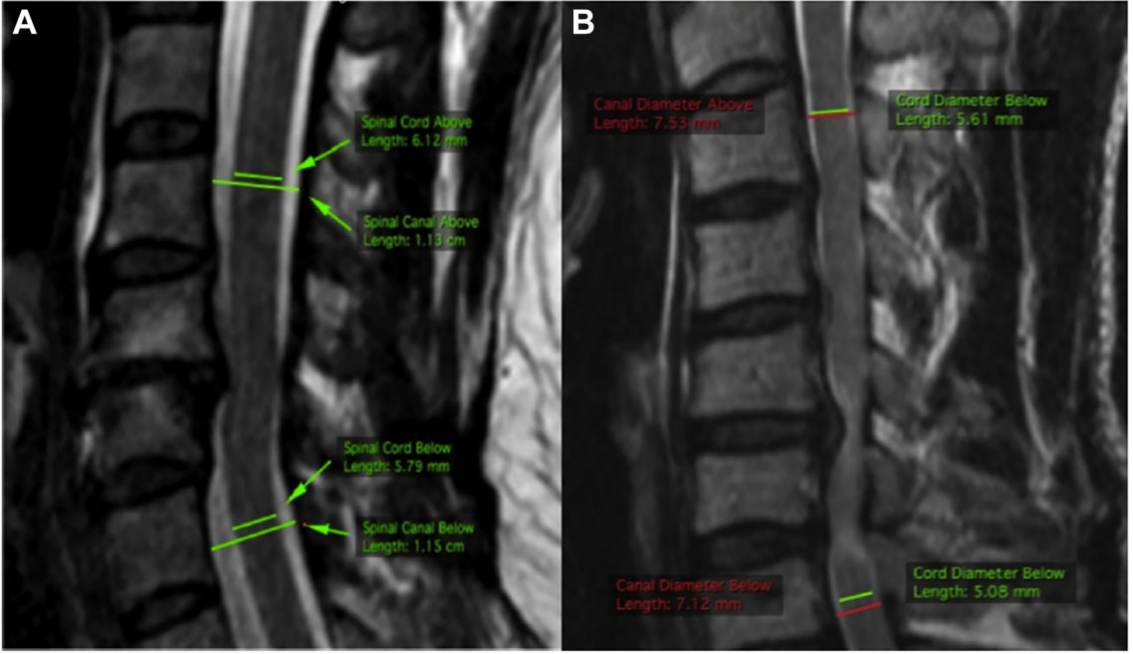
Cord-Canal mismatch measurement in 2 different patients based on sagittal T2 MRI. A, Represents a patient without a cord-canal mismatch with a SCOR calculated at 52.2% ([6.12 + 5.79]/[11.3 + 11.5]) × 100. B, The patient has a cord-canal mismatch as evidenced by an SCOR of 73.0% ([5.61 + 5.08]/[7.53 + 7.12]) × 100. Taken from Nouri et al.^
